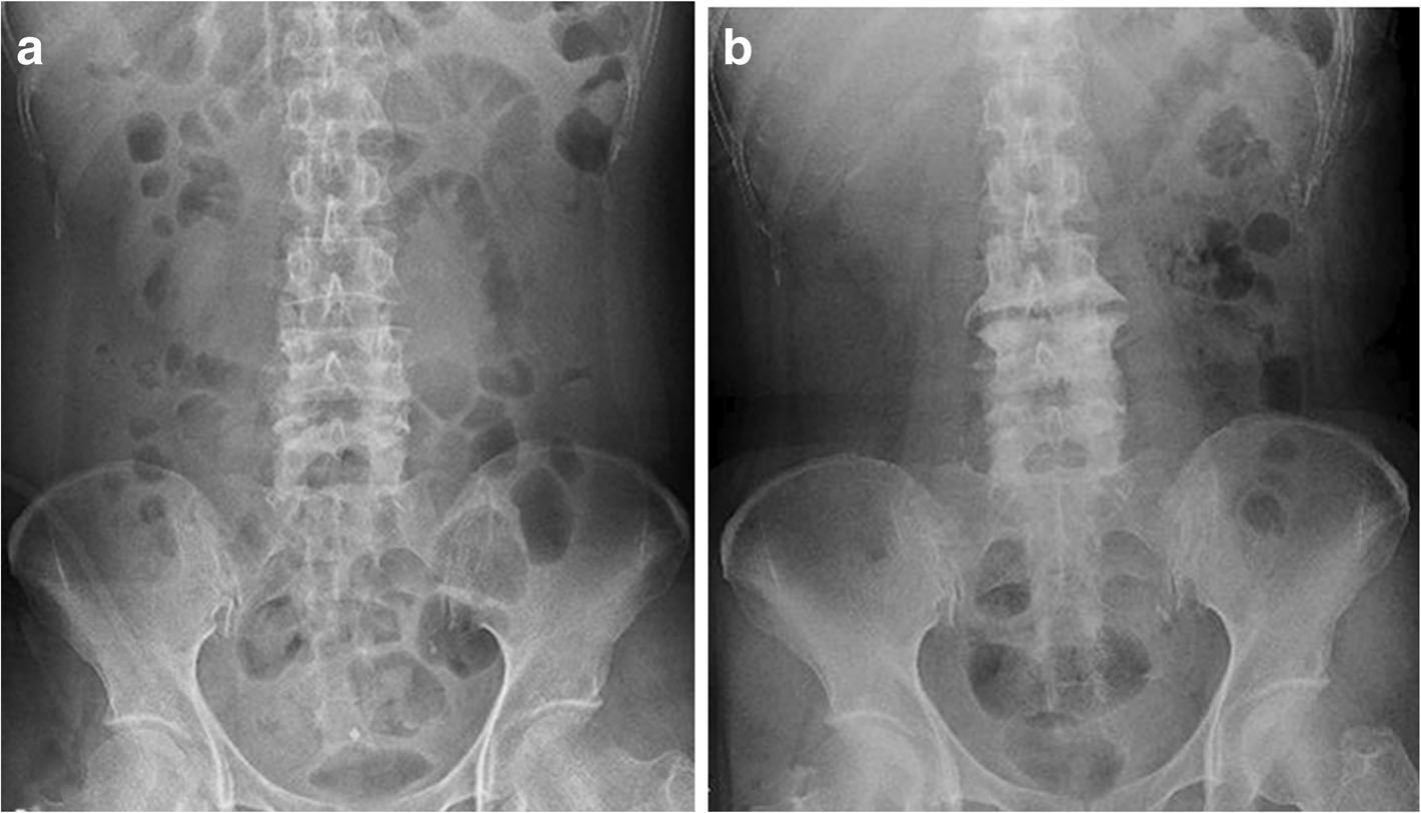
Plain radiographs show the degenerative change of the lumbar vertebra. Posteroanterior plain radiographs a before treatment and b at the final follow-up show the progression of degenerative change between the third and fourth lumbar vertebra
